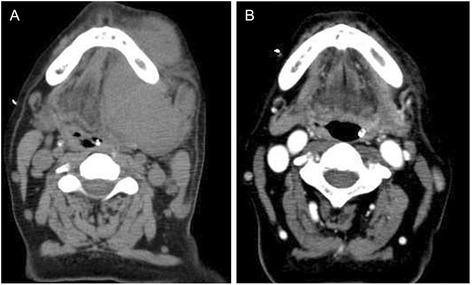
Transverse CT scan of the oral cavity showing an oral tumor at diagnosis (a) and 30 days after the initiation of bortezomib treatment (b)
